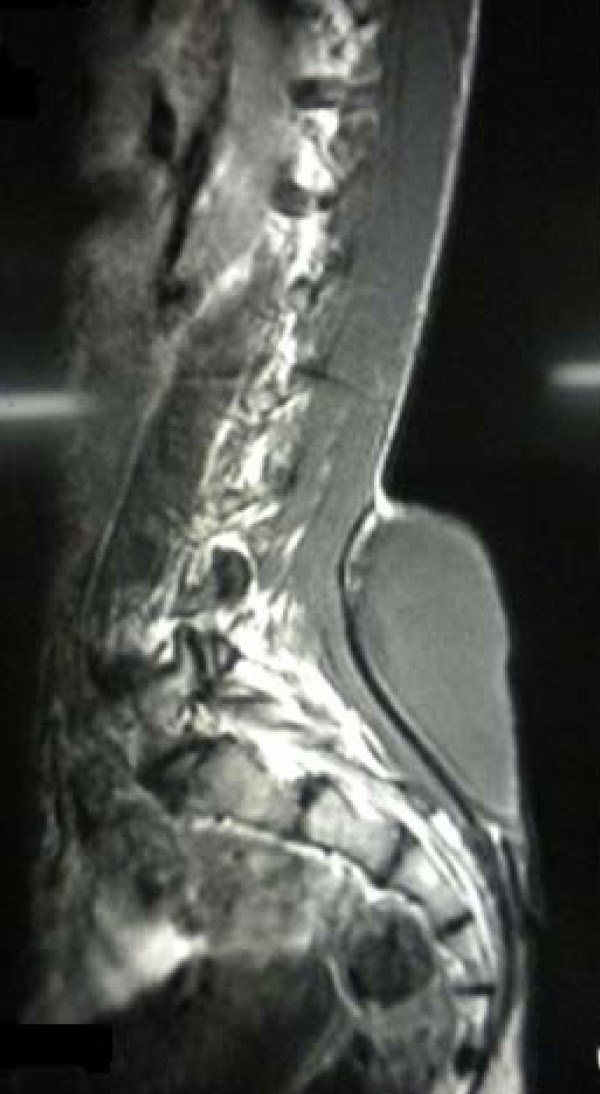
MRI scan showing the teratoma at the level of the lumbosacral region.
